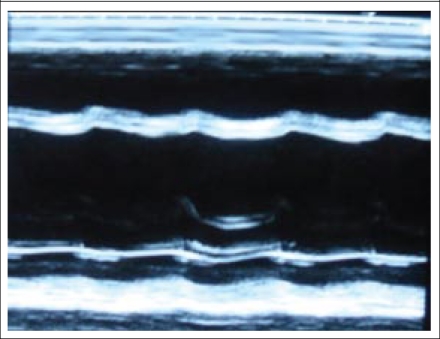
M-mode echocardiogram showing dilated left ventricle and reduced left ventricle systolic function
